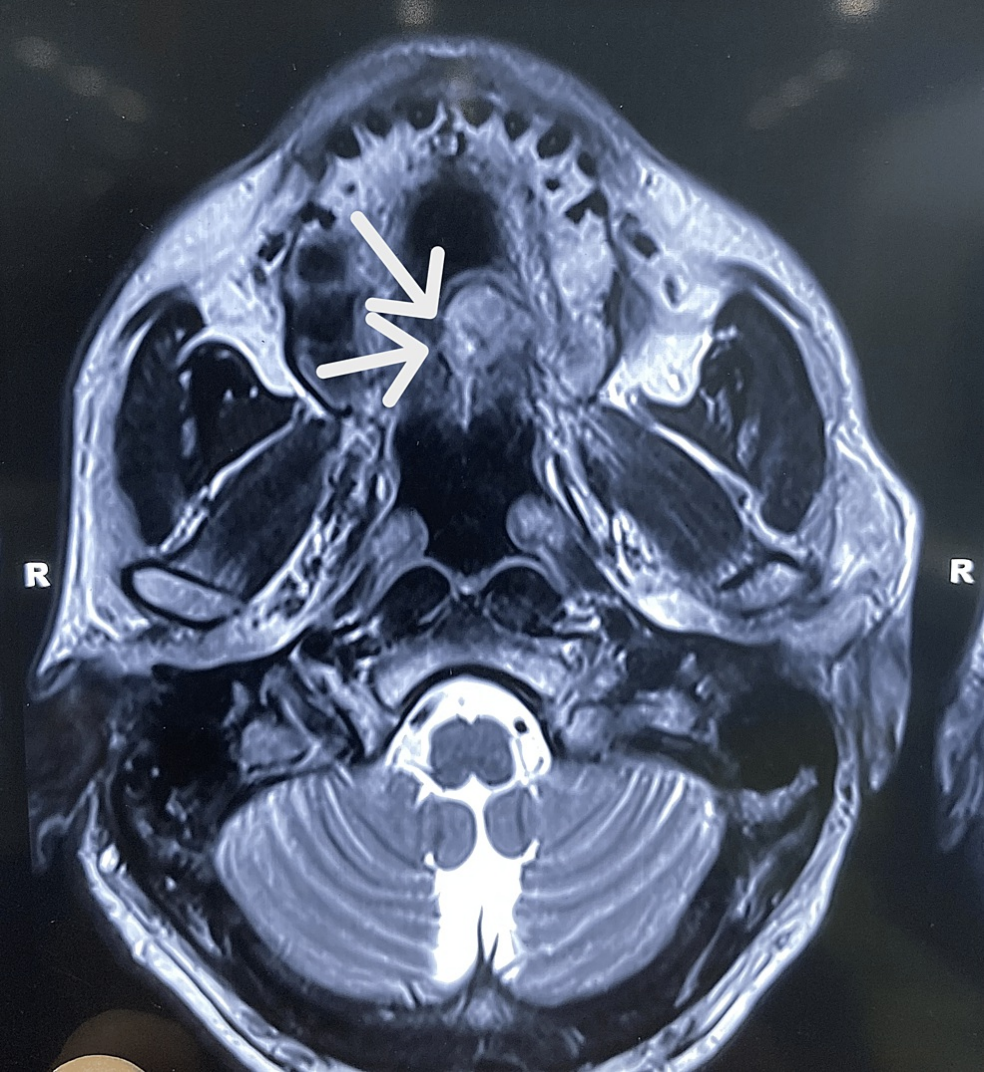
Radiograph showing the lesion in the palate The arrows in the contrast CT show a well-defined lesion on the left side of the palate.
